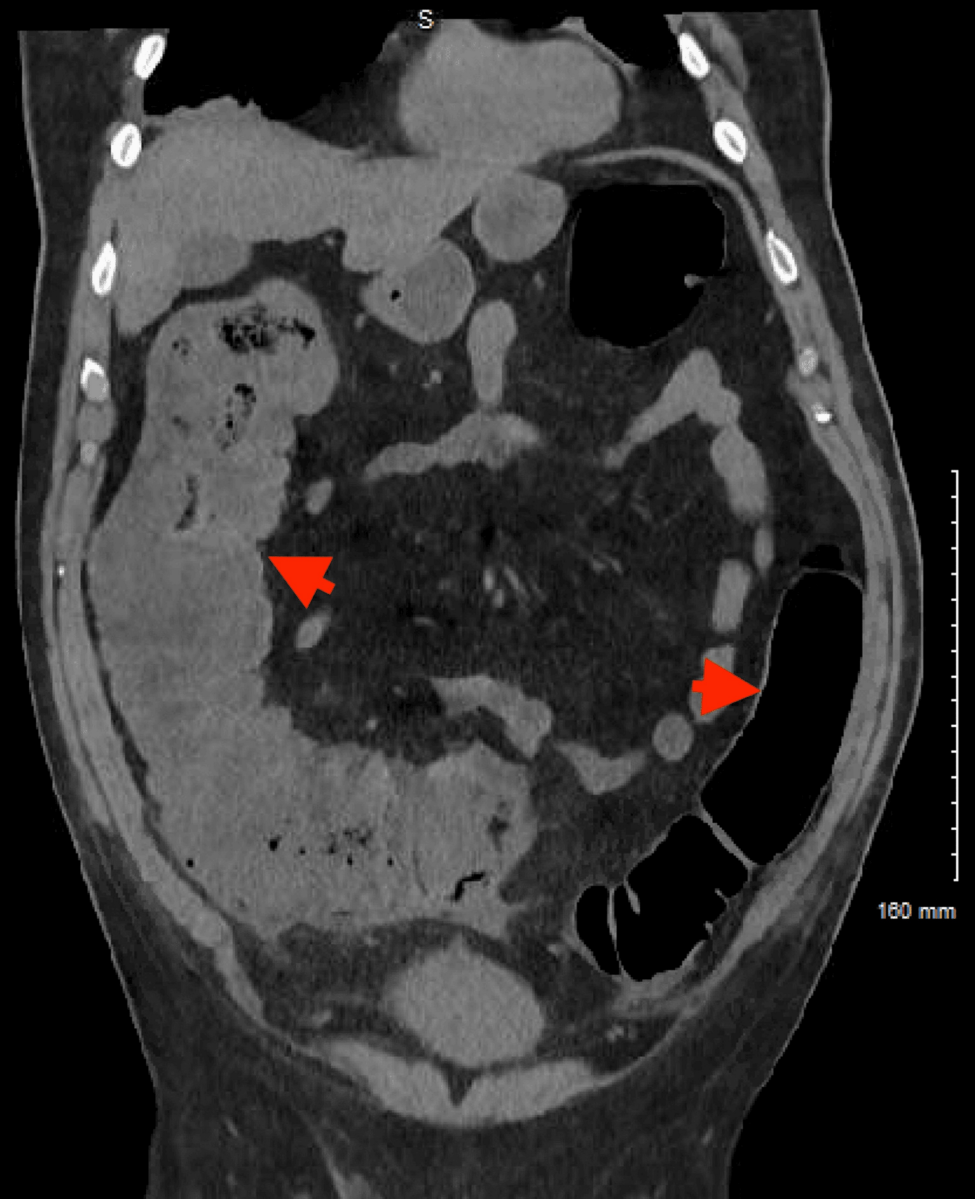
Abdominal CT scan CT scan of the abdomen confirming the colonic dilation and ileus (red arrowheads)
